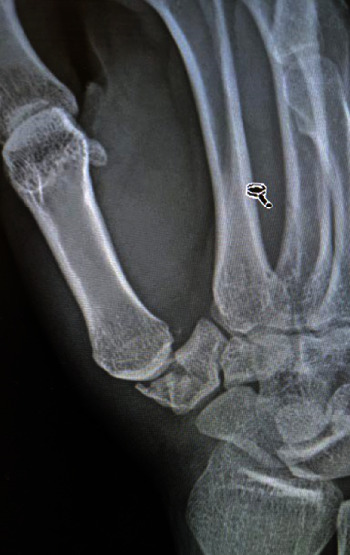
Plan radiograph at presentation demonstrating trapezium fracture with associated carpometacarpal subluxation.
